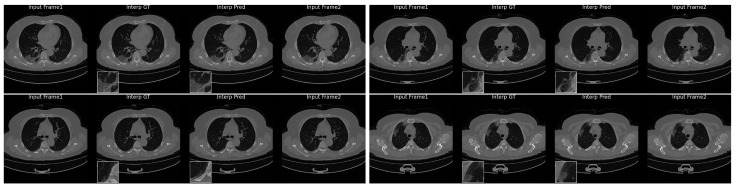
Qualitative visualization of COVID-19 CT inter-slice interpolation.
